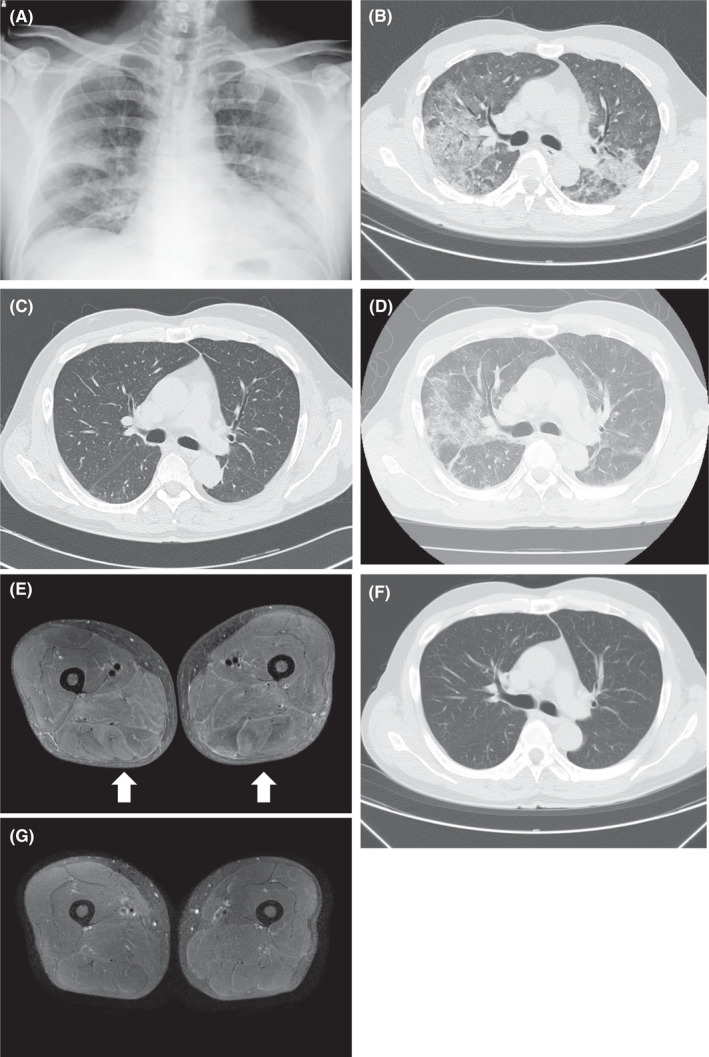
Radiologic findings of the patient. Chest X‐ray (A) and plain chest computed tomography (CT) (B) images taken on the first day of admission showed diffuse ground‐glass opacification and consolidation in bilateral lung fields. (C) A plain chest CT image taken on the 13th day of the first admission showed the disappearance of abnormal shadows with minimal scarring. (D) A plain chest CT image taken on the first day of the 2nd admission demonstrated a relapse of diffuse ground‐glass opacification and consolidation in bilateral lung fields. (E) The short‐tau inversion recovery (STIR) of magnetic resonance imaging (MRI) on the 3rd day of the 2nd admission showed a high signal in the hamstring muscles (*arrows*), which reflects muscle edema and inflammation. (F) A plain chest CT image on the 17th day of the 2nd admission showed resolution of abnormal shadows. (G) The STIR of MRI taken on Day 24 of the second admission showed resolution of high signal in the hamstring muscles
